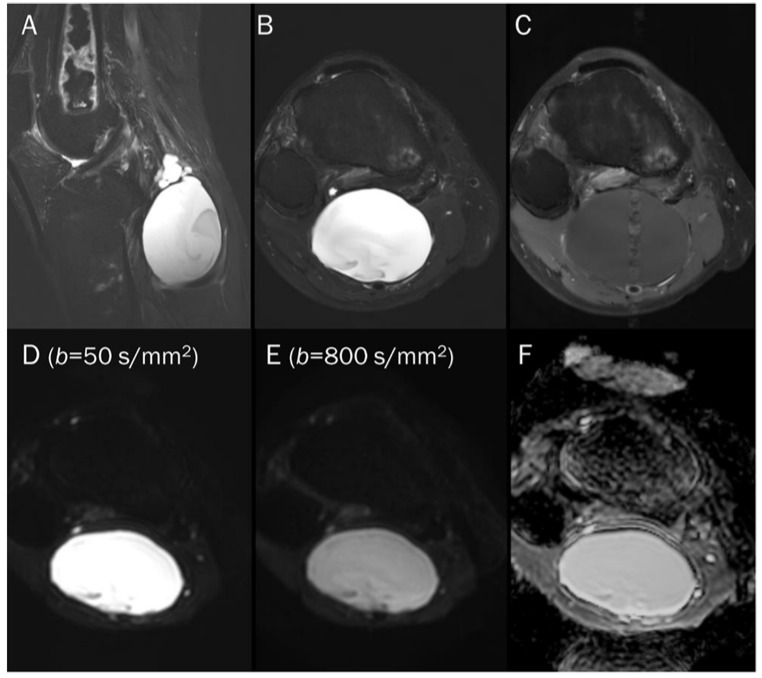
A 55-year-old male with a ganglion cyst in the popliteal fossa. (A) Sagittal and (B) axial T2-weighted fat-suppressed images show a mass with a bright signal intensity mass in the popliteal fossa. (C) Axial T1-weighted enhanced image shows peripheral thin wall enhancement. (D,E) DWI with *b*-values of 50 and 800 s/mm^2^ exhibit persistent hyperintensity with (F) no reduction in the ADC map, suggesting a T2 “shine-through” effect, possibly due to a high T2 relaxation time.
